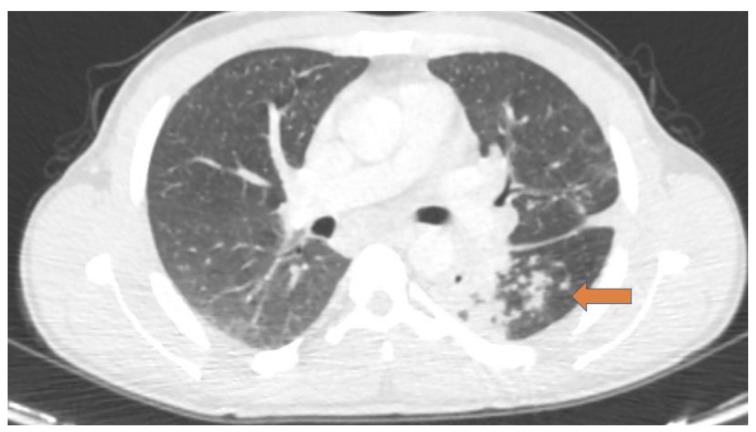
A case of Eritrean patient with chronic pulmonary schistosomiasis; axial computed tomography (CT) images showed multiple areas of consolidation in the left lung (orange arrow). A subsequent lung biopsy demonstrated parenchymal granulomas with associated schistosome eggs.
